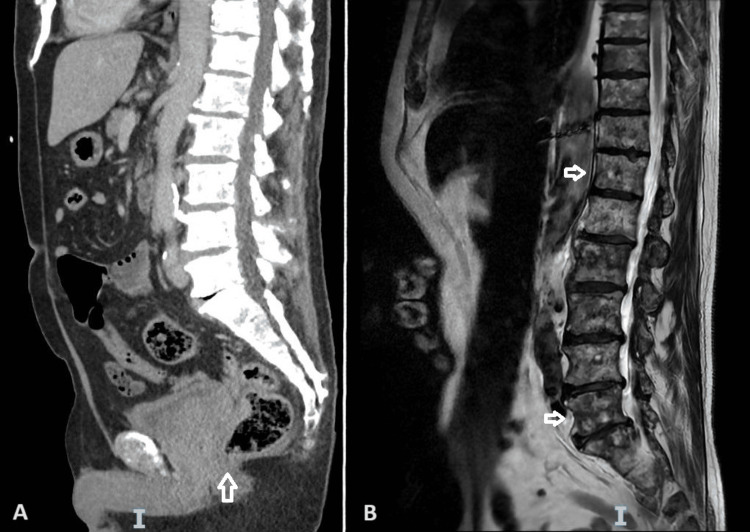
Sagittal views of staging CT scan of the abdomen and pelvis, as well as MRI of the lumbosacral spine. A: Sagittal view of staging CT scan (chest, abdomen, and pelvis) clearly showing irregular appearances of the prostate gland with loss of adjacent fat planes and tumour extension into the seminal vesicle and lower rectum (as indicated by white arrow). B: Sagittal view of MRI lumbosacral spine showing diffuse skeletal metastases with multiple lytic and sclerotic lesions (as indicated by white arrows) but no evidence of cord compression. CT: computed tomography; MRI: magnetic resonance imaging
